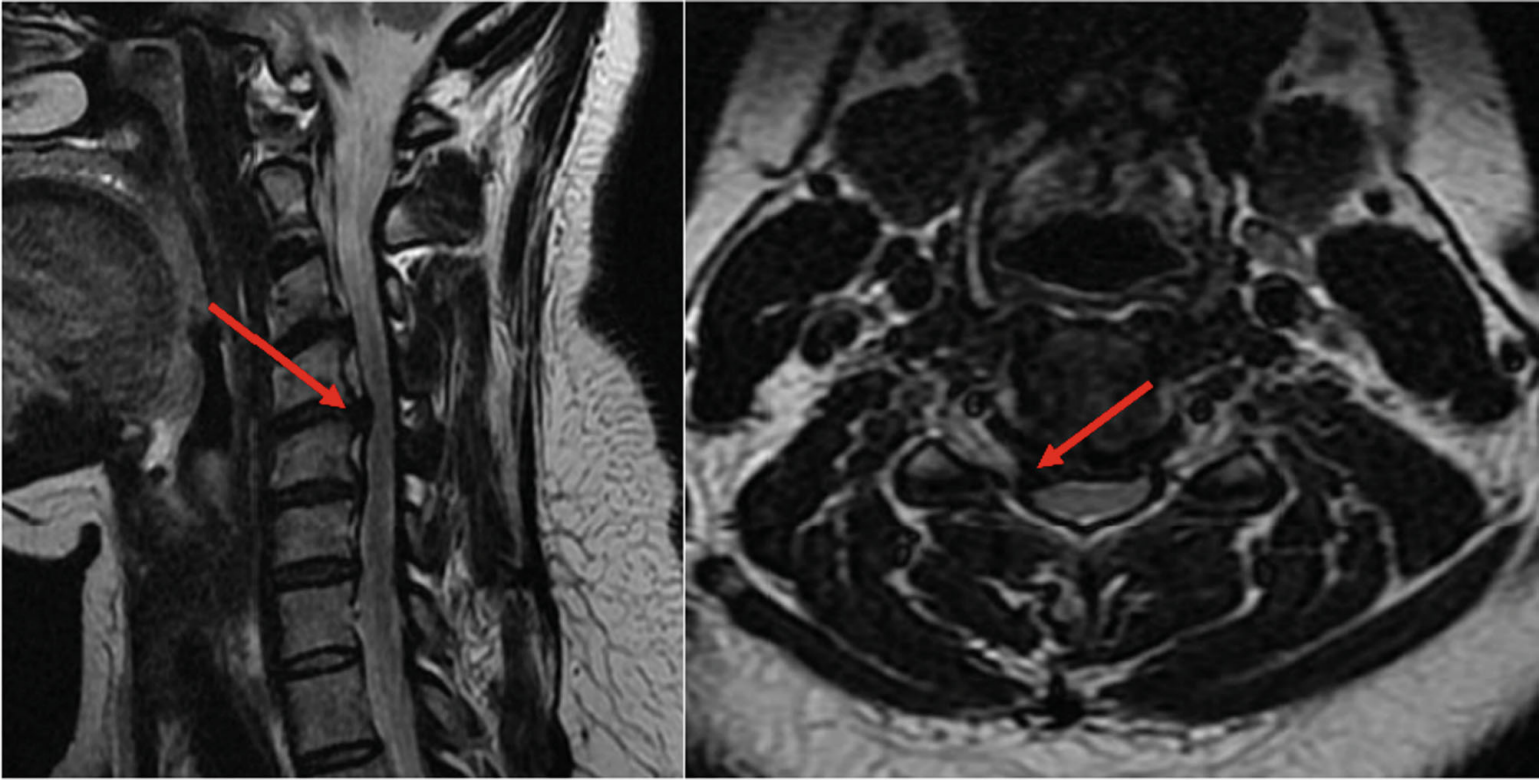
Cervical MRI (sagittal and axial sections) showing C4–C5 disc herniation and right foraminal stenosis.
